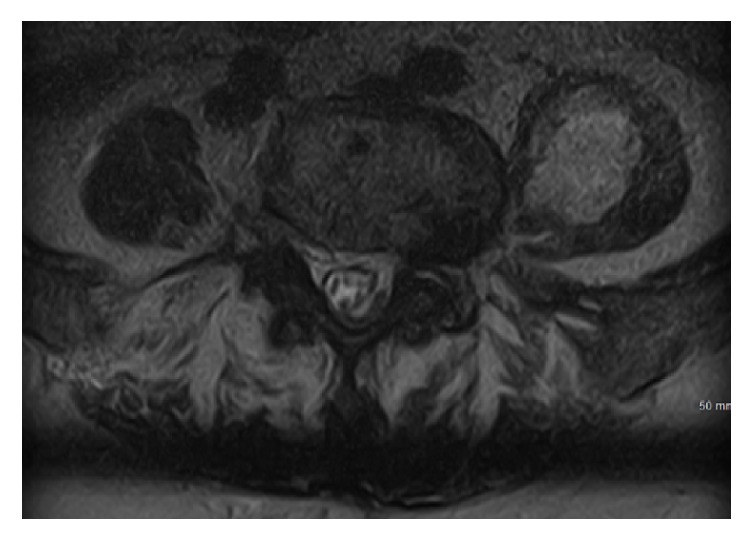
Repeat MRI of the lumbar spine on hospital day 10. Visible here is a persistent fluid collection within the left psoas muscle that has increased in size from previous MRI (Figure 2) despite CT guided aspiration.
